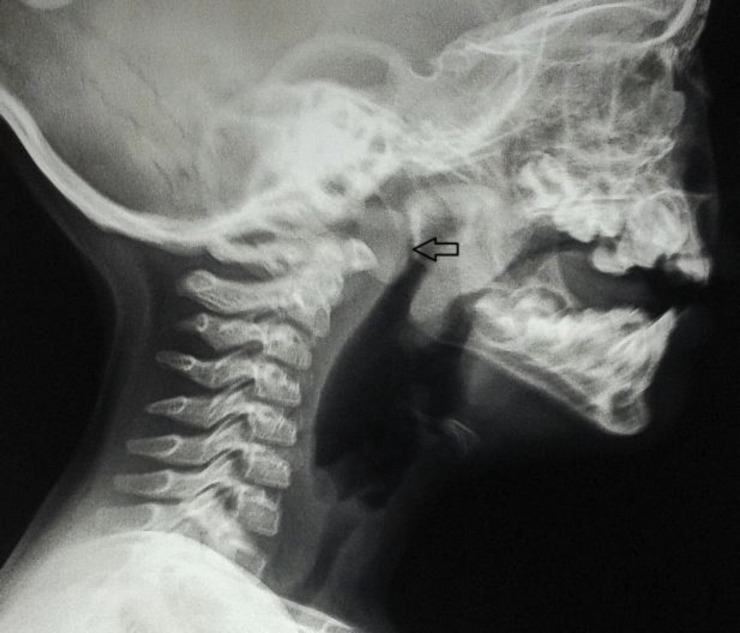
X-ray showing the round, radiolucent foreign body (arrow) in the nasopharynx abutting against the soft palate.
